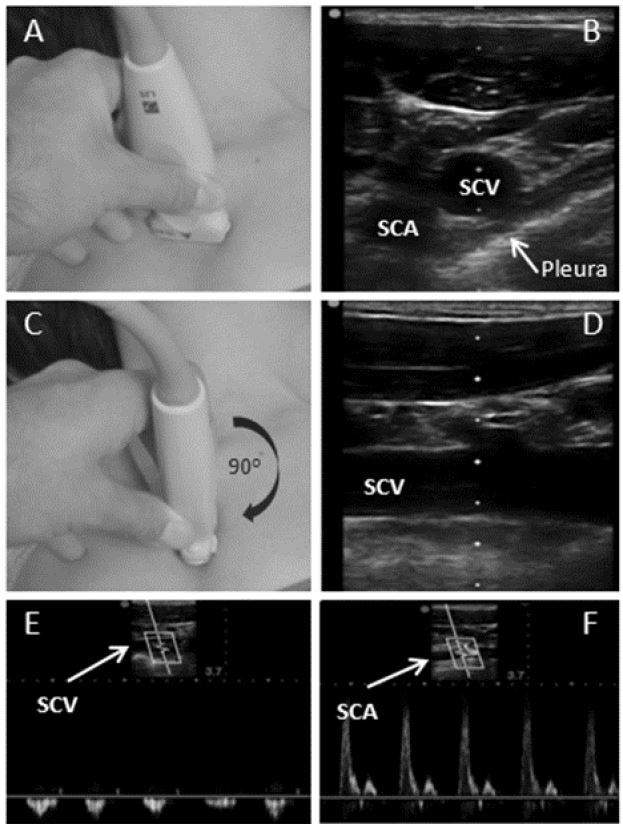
A) Linear transducer is placed perpendicularly and inferior to clavicle. B) Identified anatomical structures include the transverse (short axis) view of subclavian vein (SCV), subclavian artery (SCA) and pleura. C) With SCV centrally positioned, the transducer is rotated 90° clockwise until D) longitudinal view of subclavian vein is obtained. E) Pulse-wave Doppler view of the SCV confirms non-pulsatile flow and identifies the vessel. F) Tilting the transducer cephalad enables the visualization and identification of SCA with pulse-wave Doppler ultrasound for better anatomic orientation.
